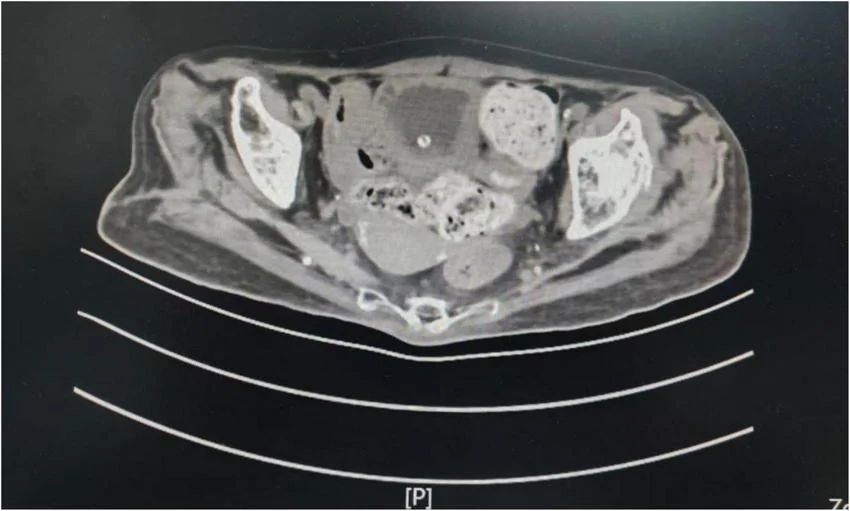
Thickened bladder wall without gas shadows on the re-examined abdominal CT scans on August 17, 2025.
